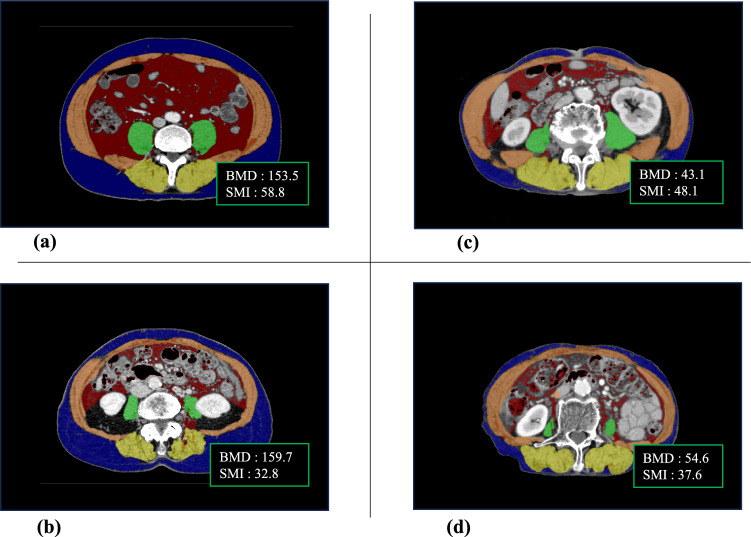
Representative computed tomography images showing body composition ranges. Ranges of body composition include within normal (a), with sarcopenia—decreased skeletal muscle index (b), with osteopenia—decreased bone mineral density (c), and with osteosarcopenia—decreased skeletal muscle index and bone mineral density (d).
